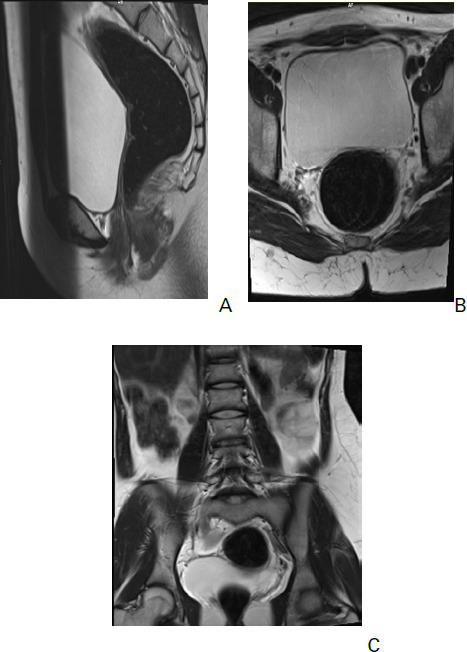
Sagittal and axial T2 weighted MR images show absence of uterus within the pelvic cavity (A) and (B). Coronal T2 weighted MRI shows absence of kidney in bilateral renal fossa (C).
